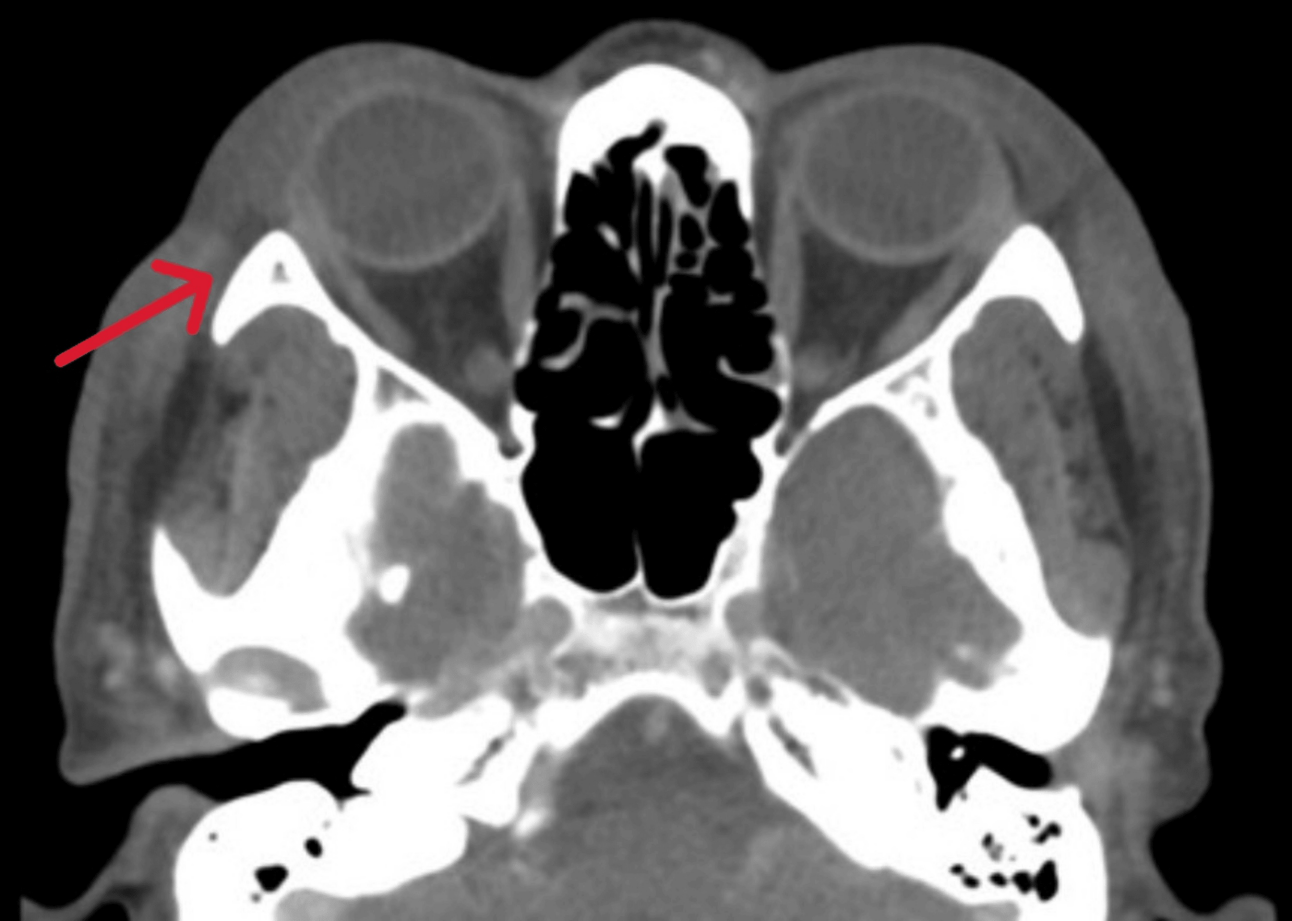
CT scan axial view revealed right periorbital (pre-septal) soft tissue thickening (red arrow).
